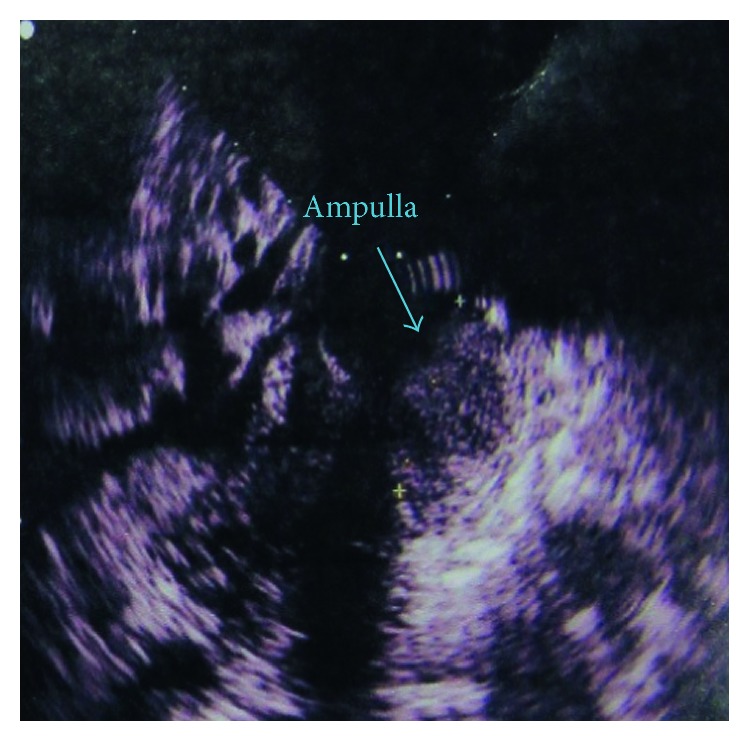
Endosonographic imaging exhibited a hypoechoic lesion at the ampulla.
